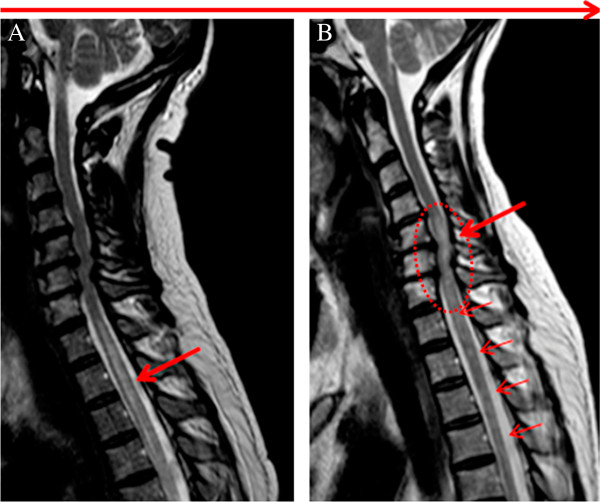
Modifications of longitudinally extensive transverse myelitis (LETM). Spinal cord MRI: sagittal T2WI of spinal cord from an anti-AQP4 antibody positive patient with NMO A: primary LETM in the upper thoracic cord (arrow) extending from Th1 – 6 (lower limit not shown) B: Fragmentation (small arrows) of the earlier LETM following treatment with high-dose steroids and a new LETM (circle) in the lower cervical cord 3 months later.
